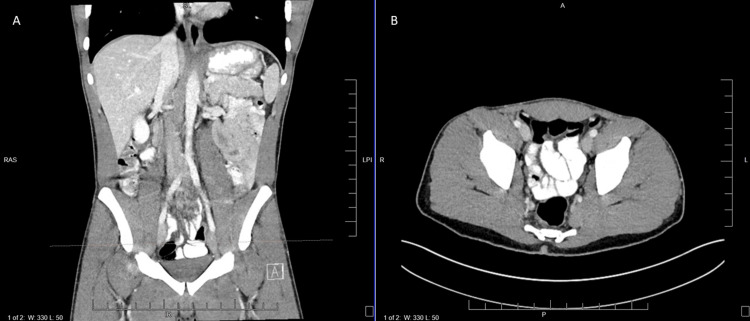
CT abdomen and pelvis with contrast from January 1, 2023 A) CT abdomen and pelvis coronal view. The dotted orange line indicates the corresponding level in Panel B. B) CT abdomen and pelvis axial view.
